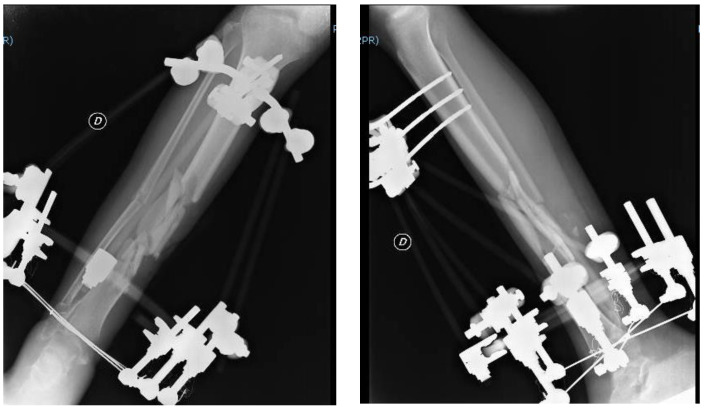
Radiograph at the three-month follow-up evaluation; AP and LL projections of the right leg and ankle.
